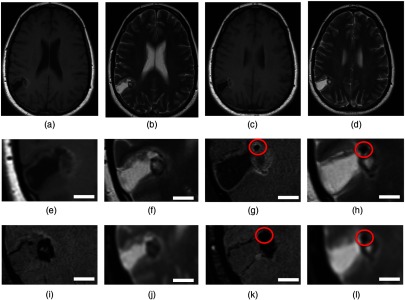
In vivo* MRI findings. Representative images from two adjacent slices with the lesion on (a and c) T1-weighted image and (b and d) T2-weighted image in the upper panels. The magnified views of the lesion with each MRI sequence are shown in the lower panels: (e and g) T1-weighted image without contrast enhancement, (f and h) T2-weighted image, (i and k) SWI, and (j and l) ADC. The scale bar indicates 10 mm in actual length. (g, h, k, and l) A nodule with MRI signals characteristic for heavily calcification is indicated in red circles.
